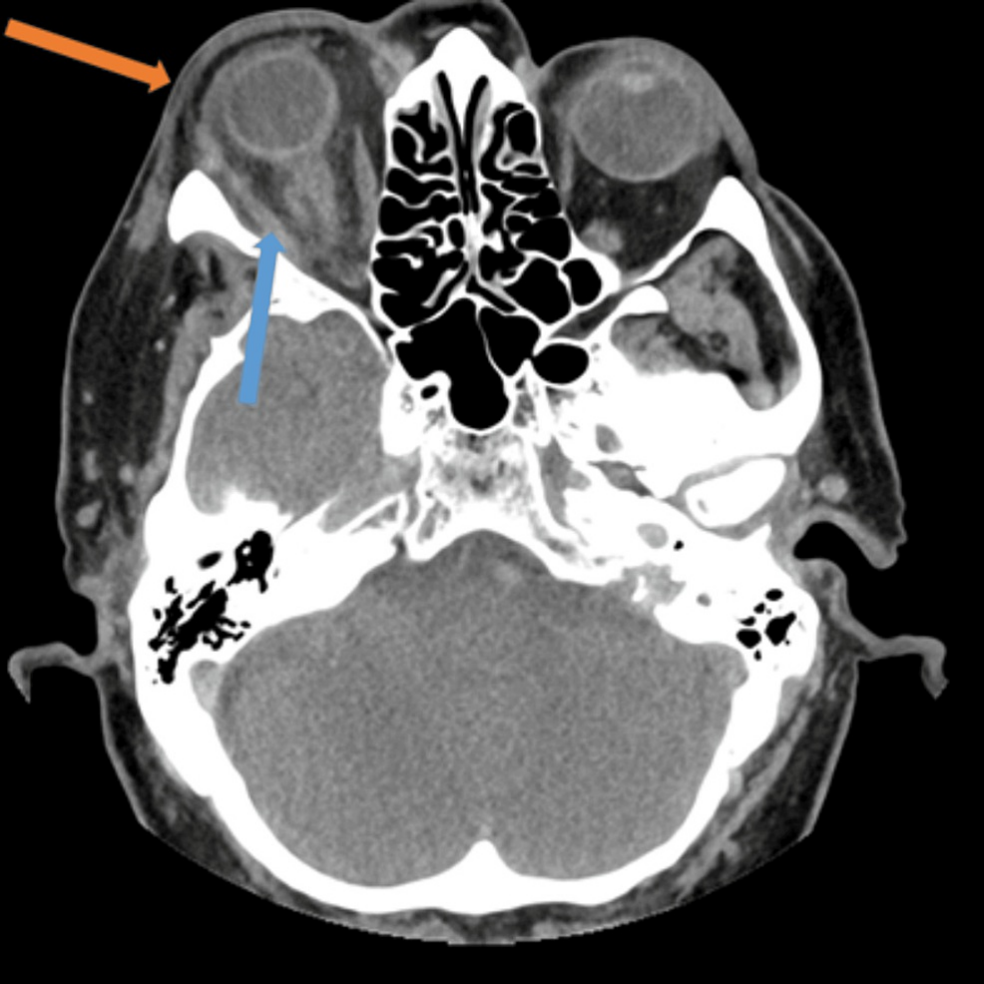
Contrast-enhanced CT orbits – axial image Periorbital soft tissue thickening (orange arrow) and oedematous extraocular muscles (blue arrow) with generalised orbital oedema. Note the presence of proptosis.
